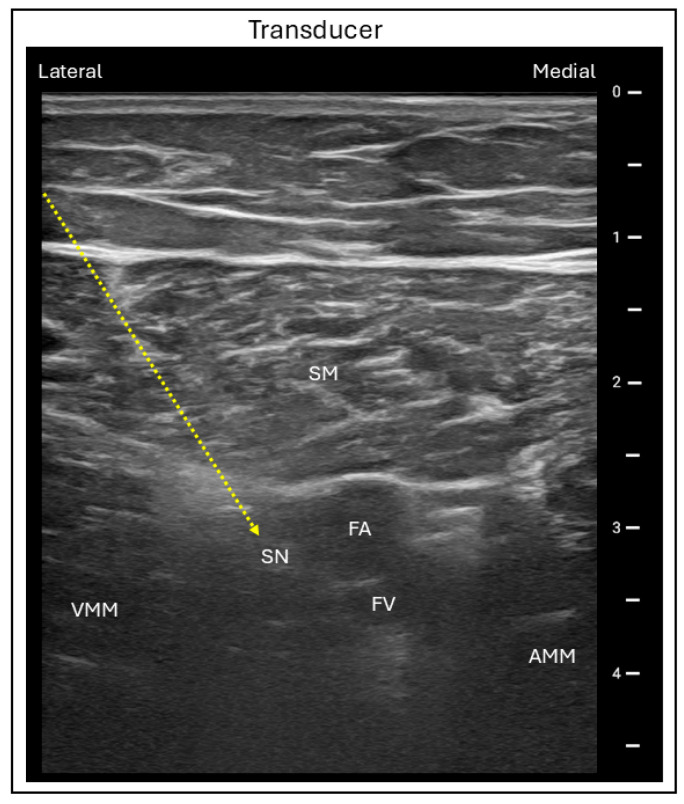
Ultrasound image demonstrating the lateral in-plane technique for adductor canal block. Dashed line = Needle trajectory; AMM = Adductor magnus muscle; SM = Sartorius muscle; VMM = Vastus medialis muscle; SN = Saphenous nerve; FA = Femoral artery; FV = Femoral vein.
